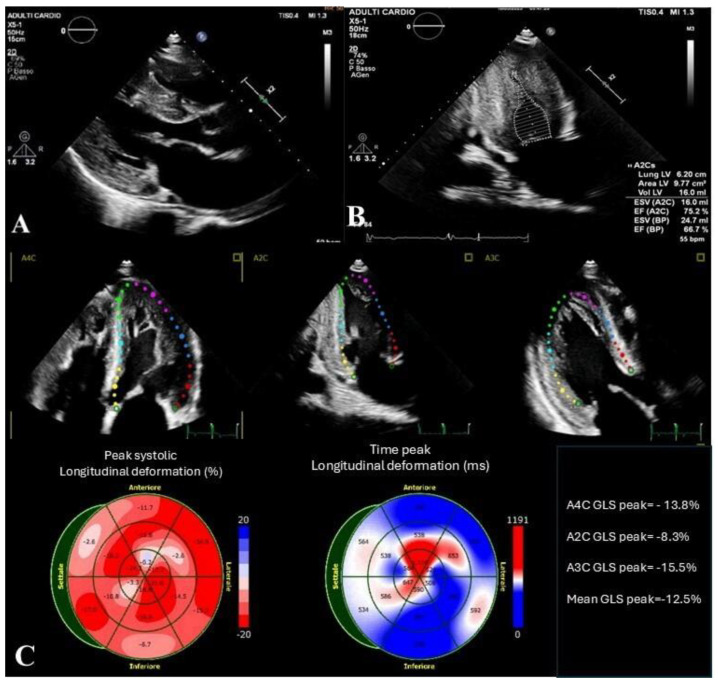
Case of a 57year-old male with Anderson–Fabry disease and coexisting sarcomeric mutations. The patient, initially presenting with mild left ventricular hypertrophy and recurrent palpitations, underwent extensive evaluation following the discovery of cornea verticillata and angiokeratomas. Genetic testing confirmed a pathogenic *GLA* gene variant, diagnostic of Anderson–Fabry disease, along with two sarcomeric variants of uncertain significance. Cardiac MRI revealed intramyocardial edema and late gadolinium enhancement (LGE), while blood tests showed elevated LysoGb3 levels. ERT with agalsidase-α was initiated, resulting in transient clinical improvement. However, the patient demonstrated progressive ventricular hypertrophy, increased cardiac mass, and a small cerebral hemorrhage on MRI. Persistent acroparesthesias and fluctuating LysoGb3 levels, despite ERT, indicated resistance to therapy. It is important to note that the sarcomeric mutations identified were of uncertain clinical significance and were unlikely to influence the AFD phenotype or the fluctuating LysoGb3 levels but may have contributed to the overall complexity of the cardiomyopathy phenotype observed. (A) Parasternal long-axis view shows severe concentric hypertrophy of the left ventricle; (B) left ventricular ejection fraction measured by the Simpson biplane method is preserved; (C) a reduction is detected in the GLS values.
